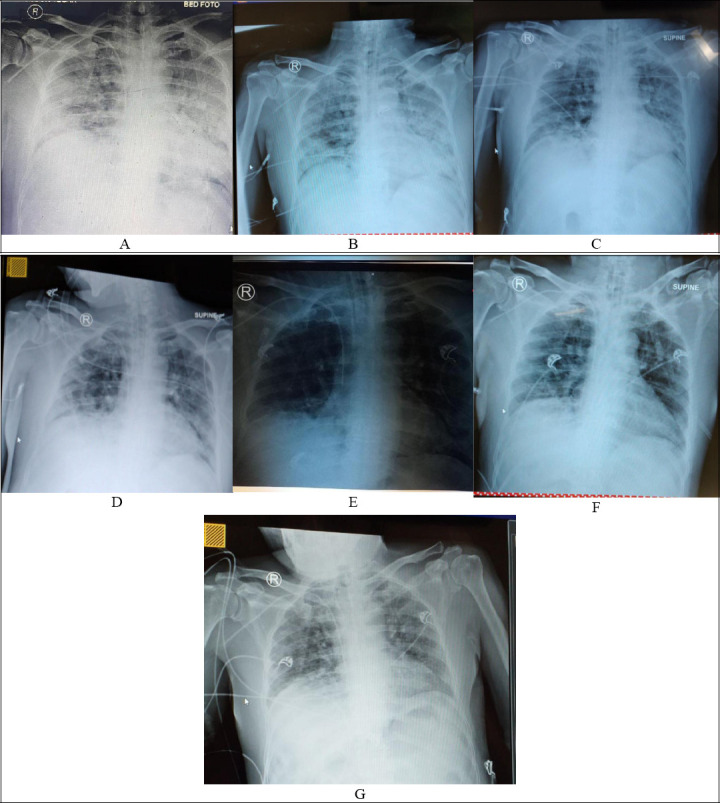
Serial Chest X-ray. A. Oct 6^th^, 2020; B. Oct 7^th^, 2020; C. Oct 10^th^, 2020; D. Oct 13^th^, 2020; E. Oct 16^th^, 2020; F. Oct 19^th^, 2020; G. Oct 22^td^, 2020.
